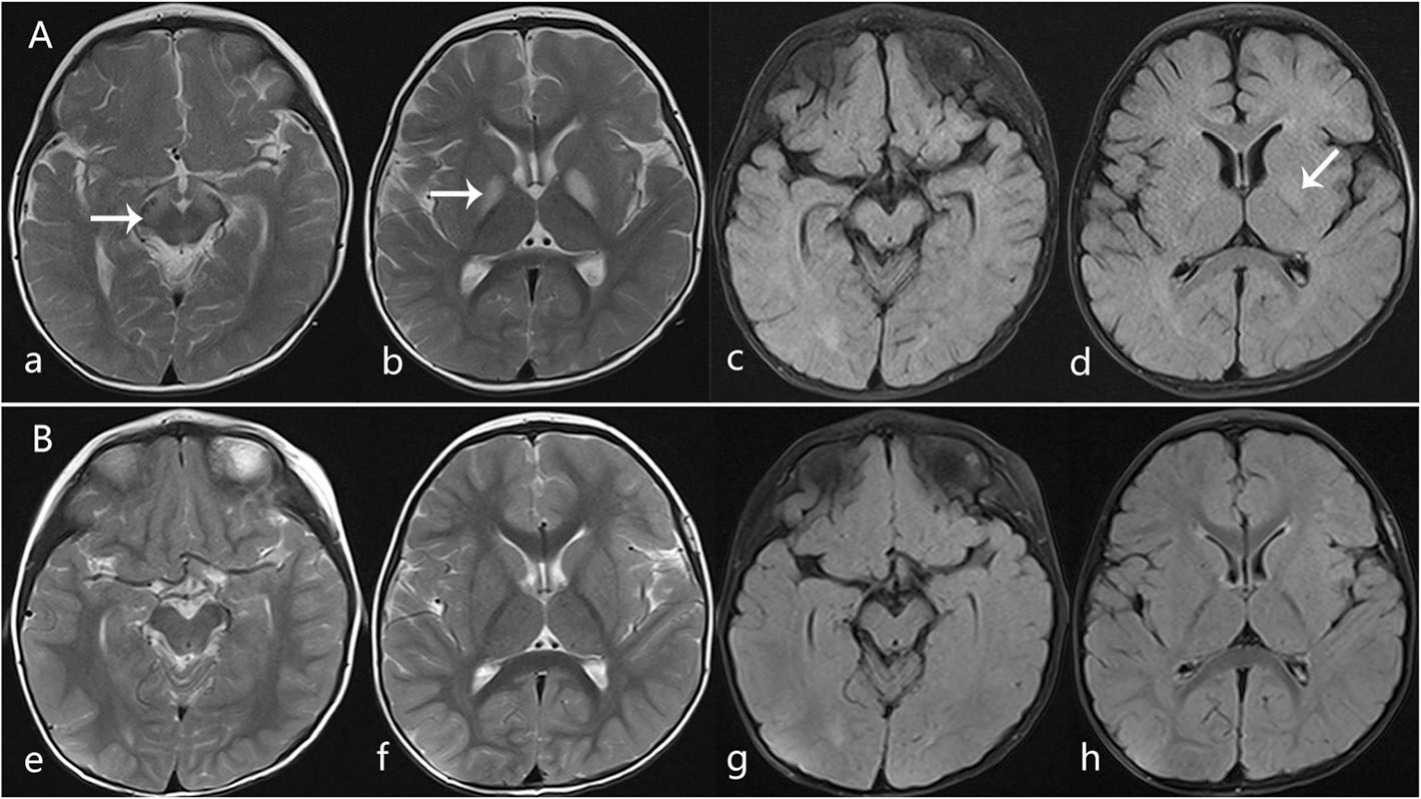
Axial T2WI and FLAIR MRI scans from Case 2. a-d, MRI abnormalities observed 2 days after disease onset at 14 months. T2WI (a, b) and FLAIR (c, d) showed high signal in the bilateral globus pallidus and substantia nigra (arrows). e-h, Follow-up images 1.5 years after onset revealed that the original lesions on the basal ganglia and substantia nigra had almost disappeared
